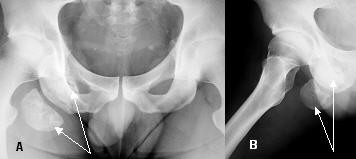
Pre-operative radiographs of the pelvis. (A) Anteroposterior; (B) lateral. The arrows point to the heterotopic bony masses.
